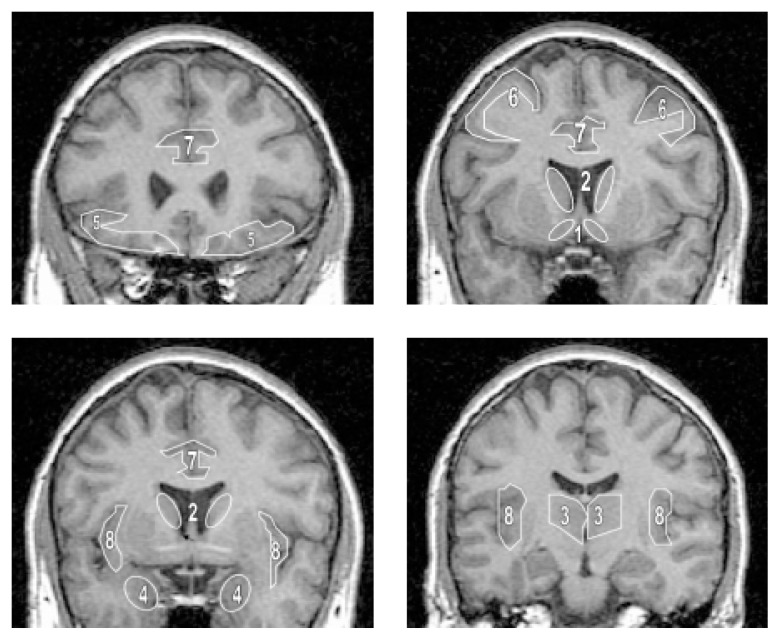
Locations of anatomical brain regions associated with craving as shown on a series of four MRI cross-sections taken from the top of the head (i.e., coronal slices). The images were generated using a T–1-weighted magnetic resonance scan of a normal human brain. This type of scan shows the brain as it usually appears in fresh or fixed post-mortem sections, with white matter appearing white, gray matter appearing gray, and the fluid surrounding the brain (i.e., the cerebrospinal fluid) appearing dark. NOTE: Numbers indicate the following brain regions: 1 = nucleus accumbens; 2 = caudate nucleus; 3 = thalamus; 4 = amygdalas; 5 = orbital prefrontal cortex; 6 = dorsolateral prefrontal cortex; 7 = anterior cingulate cortex; 8 = insular cortex. For a description of the function of these brain regions, see table 1. MRI = magnetic resonance imaging.
